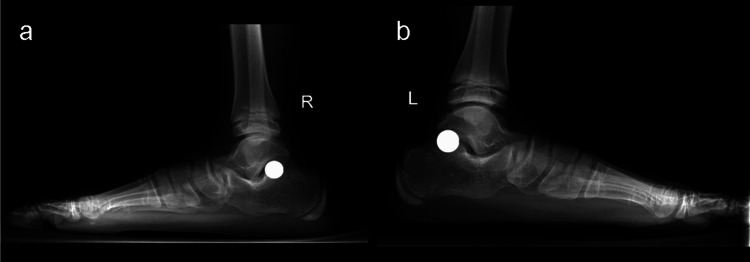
Weight-bearing lateral view plain X-rays of the right (a) and left (b) feet of the same patient in Figure 3 with painful flexible flatfoot deformity. The circular marks coincide with the position of the trigger point for pain.
